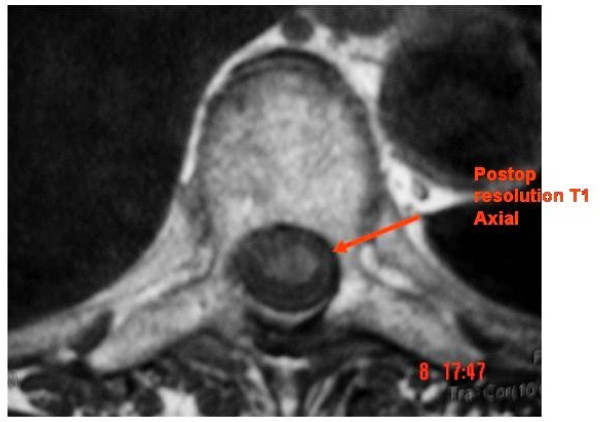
Six month post-op MRI- T1 Axial showing resolution of haematoma.
